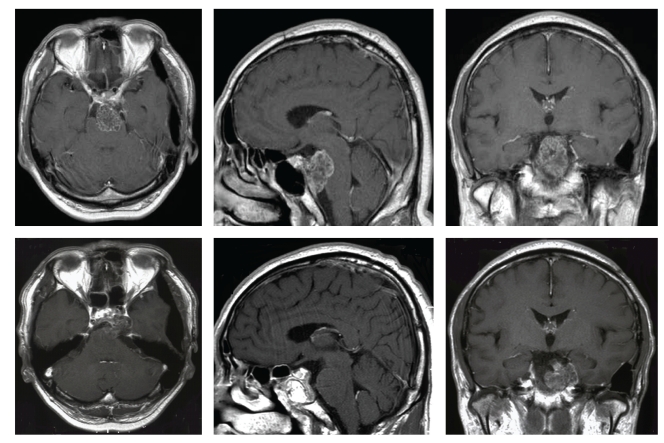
Case 1. Upper: MRI images 33 months after the first operation. The residual tumor is regrowing. Lower: MRI images after surgery, midline part of tumor was resected and decompressed brainstem.
